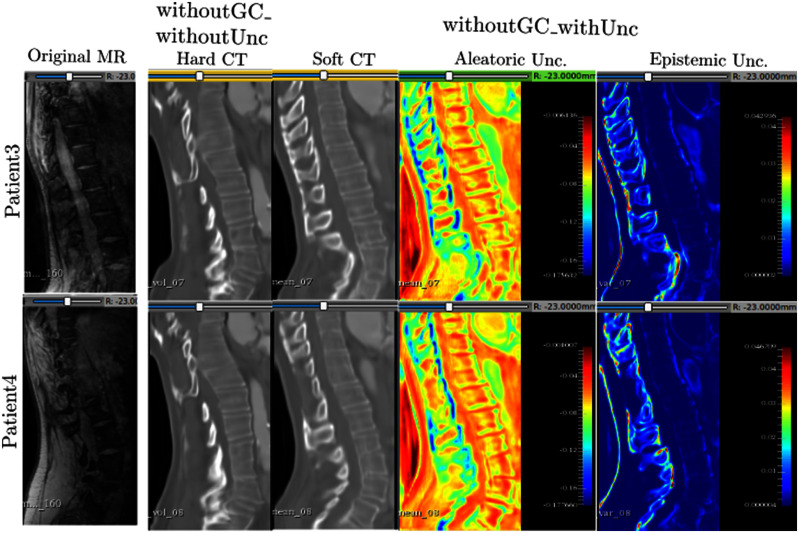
The hard and soft CT translations along with aleatoric and epistemic uncertainties for the latter. Left-to-right: Original MR slices, results for the model without uncertainty, columns 2, 3, and 4 - mean prediction with aleatoric and epistemic maps, respectively. Blue and red regions in the uncertainty maps refer to low and high uncertainties respectively. Unc. - Uncertainty.
